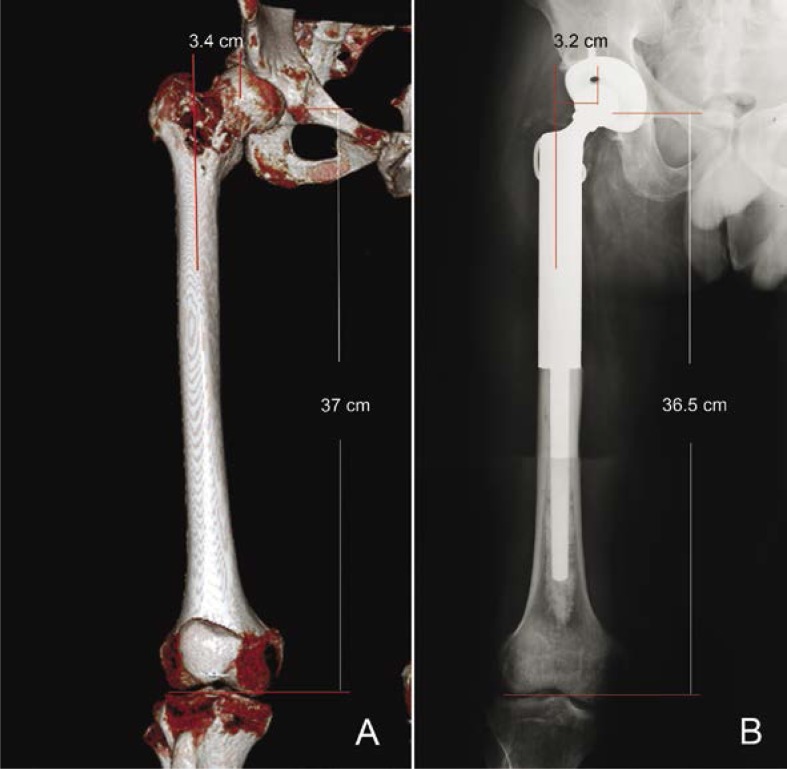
Postoperative assessment of prosthesis. A male, 31-year-old patient with chondrosarcoma in the proximal femur. Preoperative volume rendering images (A) and postoperative anterior-posterior plain film (B) demonstrate that the length and offset were accurately reconstructed.
